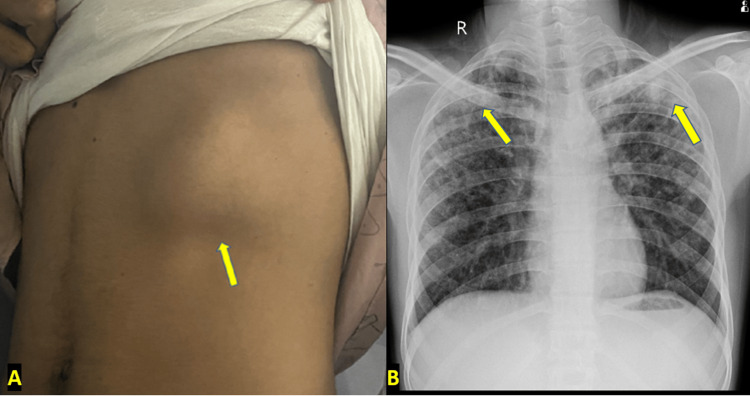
(A) Clinical image of the patient showing a swelling (depicted by arrow) over the left lower anterior chest region with no redness of the overlying skin. (B) Chest X-ray posteroanterior view of the patient showing alveolar opacities (depicted by arrows) and multiple miliary nodules in bilateral lung fields.
